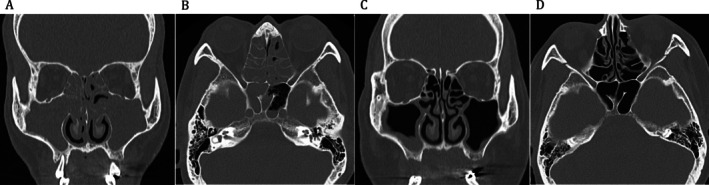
Coronal (A) and axial (B) views of computed tomography (CT) images of the sinuses at initial presentation show obstruction of the maxillary, ethmoidal, and sphenoidal sinuses. Coronal (C) and axial (D) views of CT images of the maxillary and ethmoidal sinuses 3 months after prednisolone treatment show improvement of chronic rhinosinusitis.
